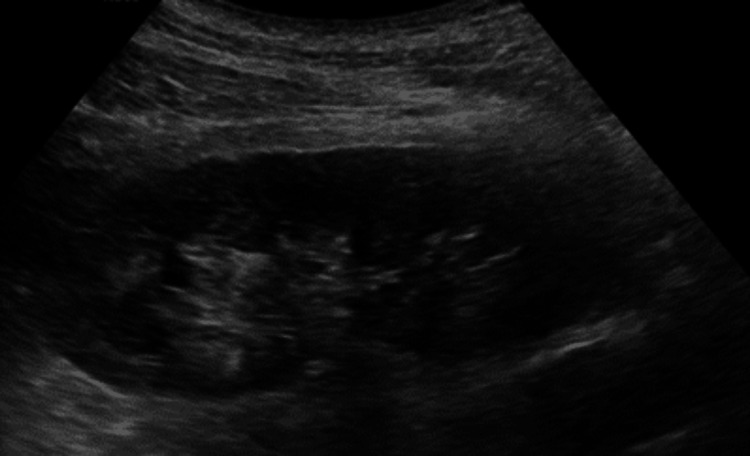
US KUB Day 134 showing complete resolution of the abscess in the left kidney. US: ultrasound; KUB: kidney, ureter, and bladder
